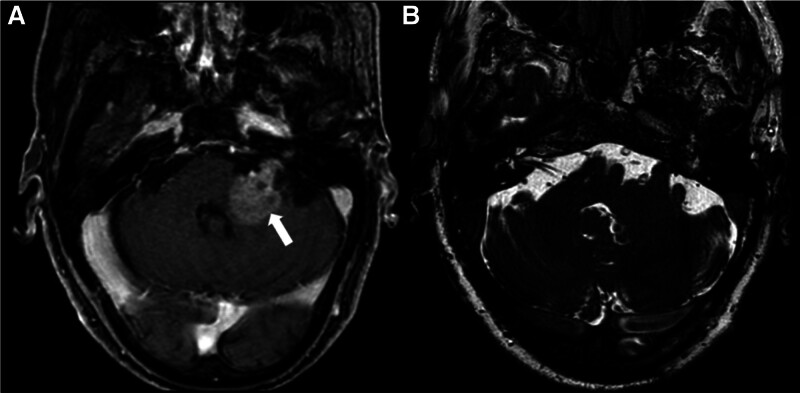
Contrast-enhanced MRI at presentation. Three-month interval MRI with (*A*) contrast-enhanced T1 sequence and (*B*) heavily weighted T2 sequence of the CPA and IAC. Contrast-enhanced T1 sequence demonstrates intra-axial mass within the middle cerebellar peduncle and CPA (white arrow). CPA indicates cerebellopontine angle; IAC, internal auditory canal.
